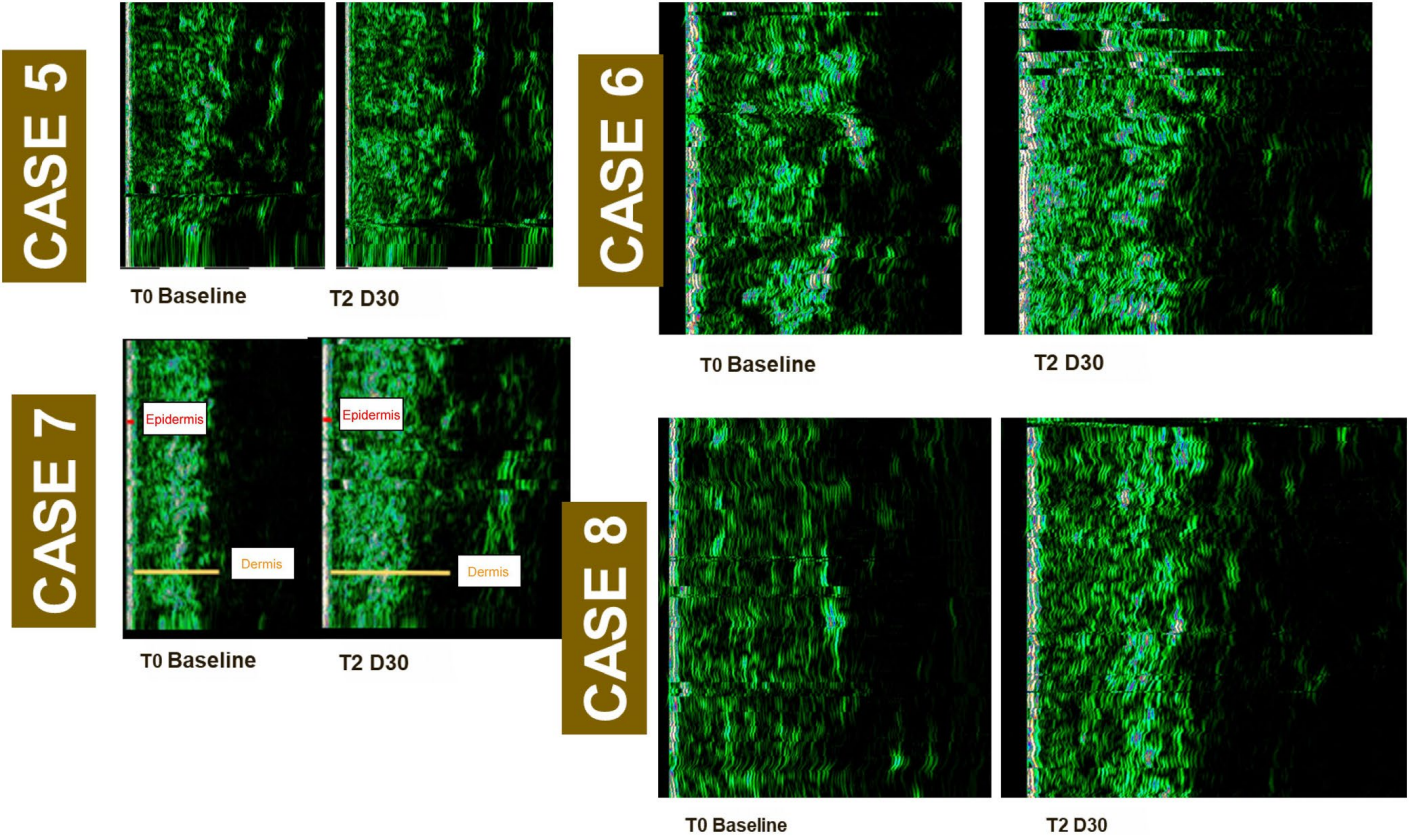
Skin ultrasound images of four cases, taken with UC22, consistent improvements in dermal density and thickness following RhCol‐III injection when comparing baseline and 90 days post‐baseline images. RhCol‐III, recombinant type III humanized collagen.
